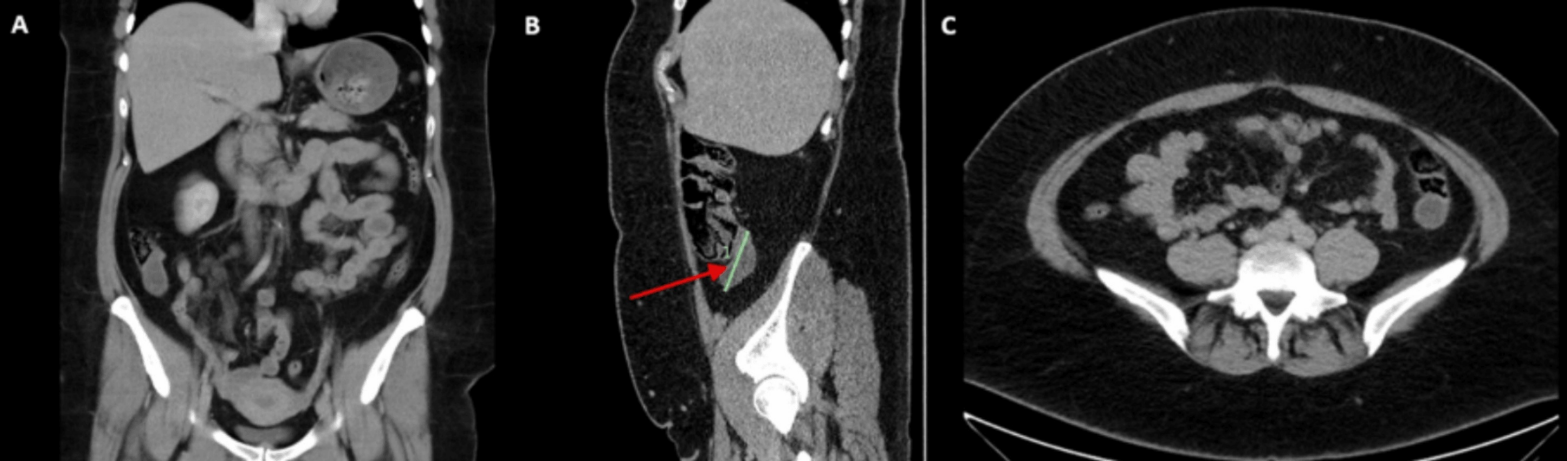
Computed tomography of the abdomen and pelvis with contrast (Case 5). (A) Coronal section; (B) sagittal section with the red arrow pointing to a tubular hypodense structure arising from the posterior cecum, posterior to the ileocecal junction and extending superiorly into the right flank, measuring approximately 6 cm in length and 2 cm in thickness; (C) sagittal section.
